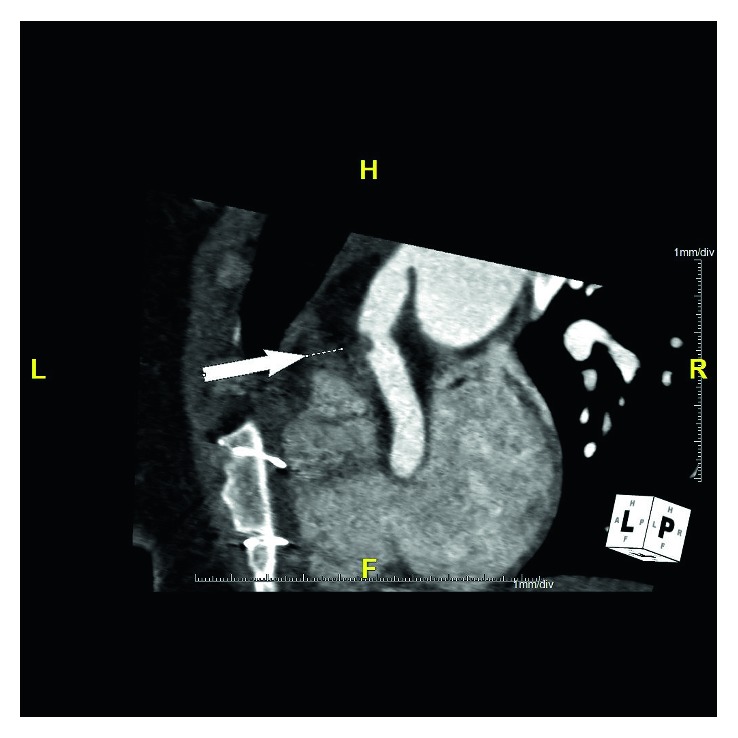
Parasagittal view of the coronary CTA performed after the patient's surgery again demonstrates the partially occlusive thrombus in the implanted RCA as pointed by the arrow.
